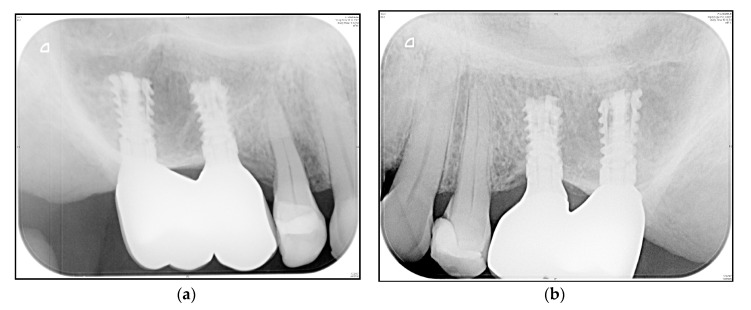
Posterior maxilla of a rehabilitated 62-year old woman at 1-year follow-up (Patient 11; Table 3). (a) Standard periapical radiogram implants i16 and i15; (b) Standard periapical radiogram implants i25 and i26.
